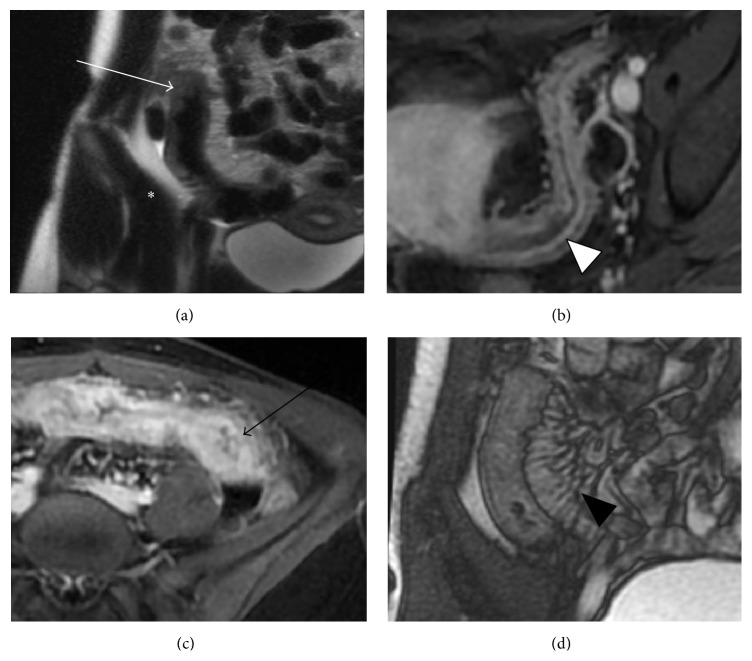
MR enterography features of active Crohn's disease. (a) Coronal T2-weighted image demonstrates wall thickening (arrow), axial T1-weighted fat-suppressed postcontrast images obtained in enteric (b) and delayed (c) phases demonstrate early mucosal ((b), arrowhead) with progressive transmural ((c), arrow) enhancement; coronal balanced steady-state free precession image (d) demonstrates mesenteric hypervascularity (arrowhead).
